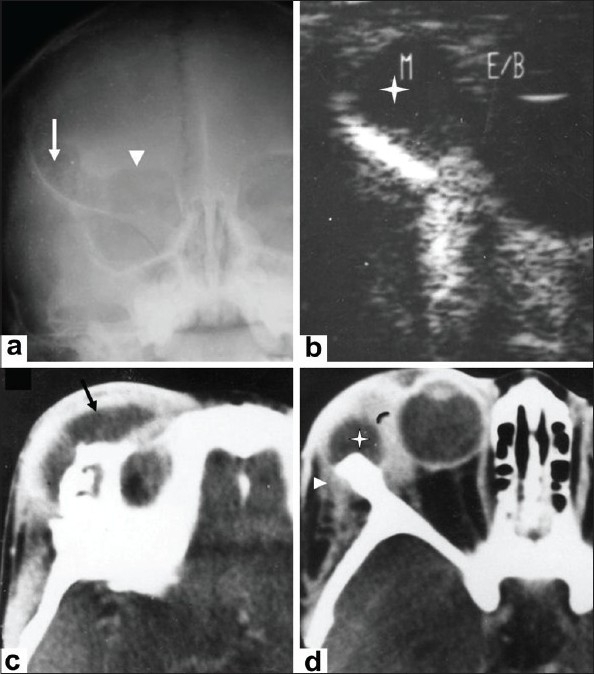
A 4-year-old boy presented with right-sided orbital swelling, with mild pain and restriction of eye movement. Caldwell view radiograph (a) shows an osteolytic lesion in the frontal bone (arrow) and destruction of the right orbital roof (arrowhead). B-scan USG of the orbit (b) shows a hypoechoic collection (asterisk) located superolaterally in the region of the lacrimal gland (lacrimal gland abscess). Axial contrast-enhanced CT scans (c,d) of the orbit, show an abscess in the lacrimal region, with rim enhancement (asterisk in d); the abscess is seen extending into the soft tissues superolaterally (causing preseptal thickening) (arrow in c) and into the infratemporal fossa (arrowhead in d).
